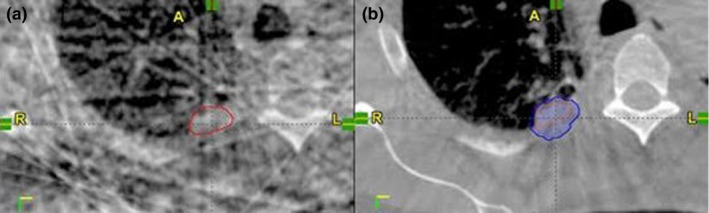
Single phase ITV (red) from patient 4 where tumor/chest wall border is indistinguishable due to significant streak artifacts in the 4DCBCT (A). Resulting 4DCBCT ITV (blue) expands posteriorly and laterally into the chest wall compared to the avgCBCT ITV (orange) and yields lower dose coverage reported to 4DCBCT ITV. This can be seen by the low Dice coefficient between avgCBCT and 4DCBCT in Table 1.
